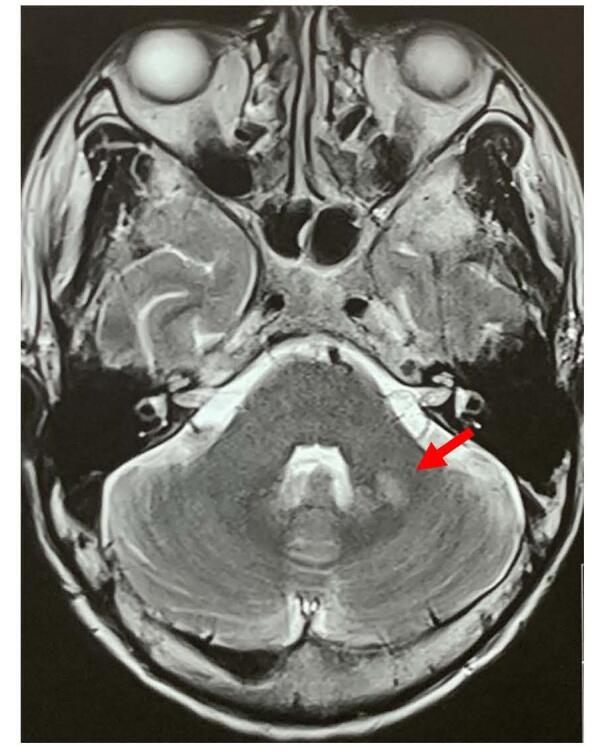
T1 weighted MRI of brain axial cut showing features of multiple tuberculomas in cerebellum (arrow).
